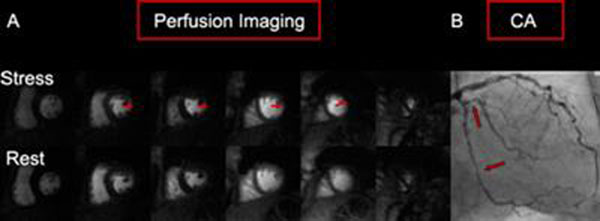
A 56-year-old man with no prior cardiac history who presented with chest pain. (A) Myocardial perfusion MRI with sliding-window conjugate-gradient HYPR (SW-CG-HYPR) detects perfusion defects in the basal, mid, and apical lateral segments, corresponding to significant stenoses in the left circumflex coronary artery (LCX). (B) Coronary angiography (CA) confirms significant stenoses in the LCX.
